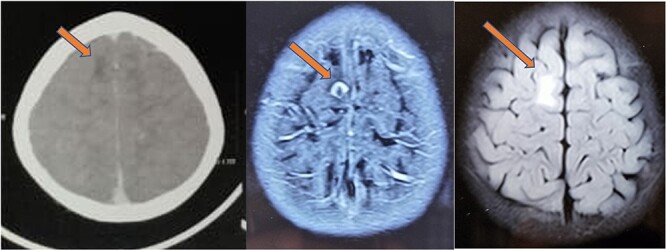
CT (left) showing a hypodense lesion in the right frontal lobe, T1 post-contrast MRI image (center) showing a ring enhancing lesion in the right frontal lobe and FLAIR image (right) showing non-enhancing vasogenic edema surrounding the lesion.
